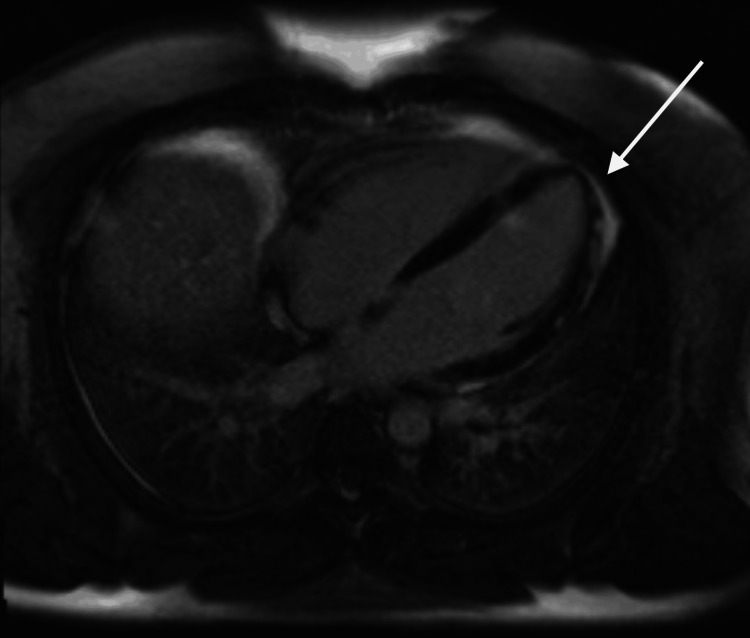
Cardiac magnetic resonance imaging showing fibronecrosis and oedema
